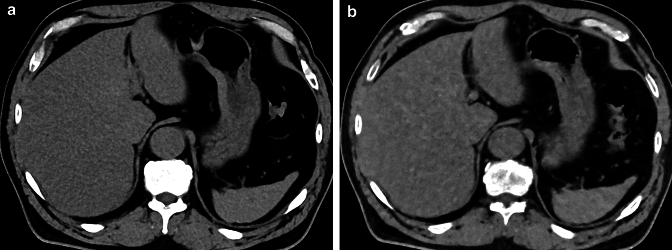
A true noncontrast CT image (A) and a virtual noncontrast CT image obtained from contrast-enhanced image data (B) acquired with photon-counting CT. Virtual noncontrast images with photon-counting CT have similar CT attenuation accuracy to true noncontrast images. In both images, the liver parenchyma is less attenuated than the spleen, indicating hepatic steatosis
